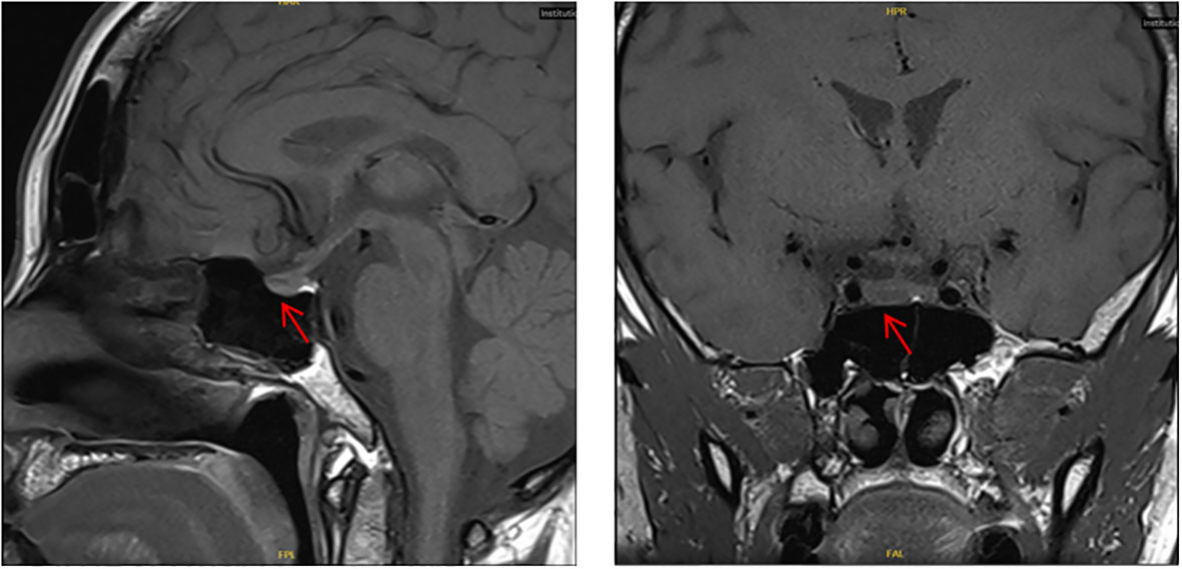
Case 1: Contrast-Enhanced MRI of the Pituitary Gland A hypointense nodular lesion is visible in the right lobe of the pituitary gland, consistent with a pituitary microadenoma.
